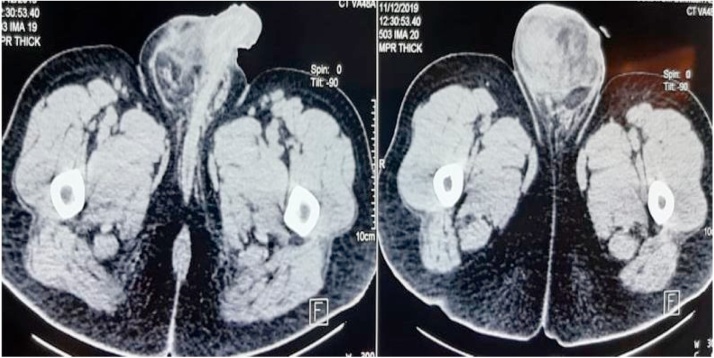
Axial CT Image showing a heterogeneously mass in the right hemi-scrotum, containing fat and soft tissue.
